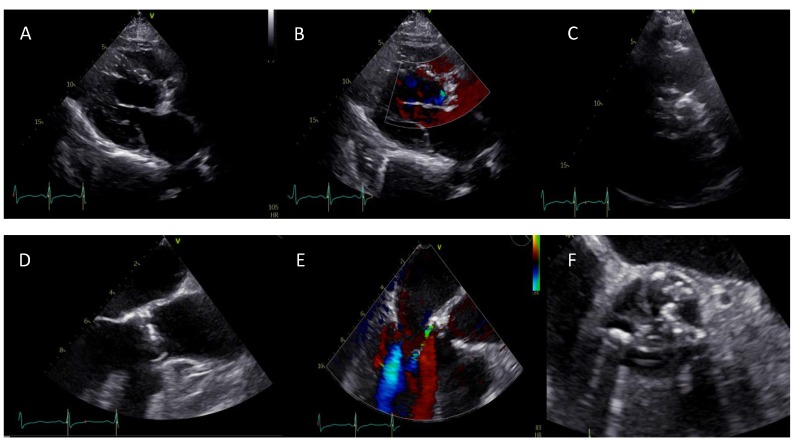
(A–C) Initial evaluation by transthoracic echocardiogram of a 49-year-old male patient. Parasternal long axis view reveals a calcified aortic valve with restricted valve opening as well as a regurgitation jet. Parasternal short axis en-face view shows a severely calcified aortic valve with a bicuspid anatomy. However, the precise type could not be identified because of the image quality. (D–F) Transesophageal echocardiography (TEE) revealed a bicuspid valve type 1 R/N morphology (Sievers) and a mild aortic regurgitation with an eccentric jet.
